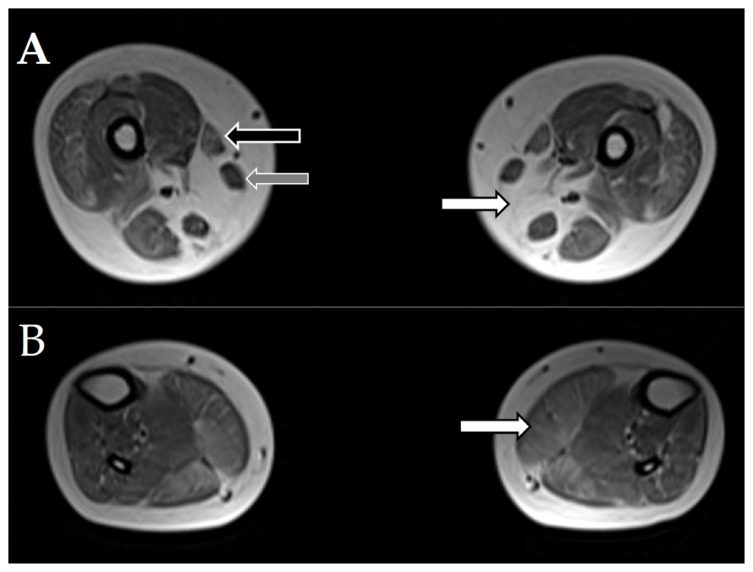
Magnetic resonance imaging (MRI) of muscles of patients 7 and 8a with *SELENON* mutations. Selected axial T1W images of the muscles. (A). White arrow points to the absence of the semimembranosus muscle. Black arrow points to the moderately affected sartorius muscle. Grey arrow points to the intact gracilis muscle. (B). White arrow points to moderate, diffuse changes in the gastrocnemius muscle.
